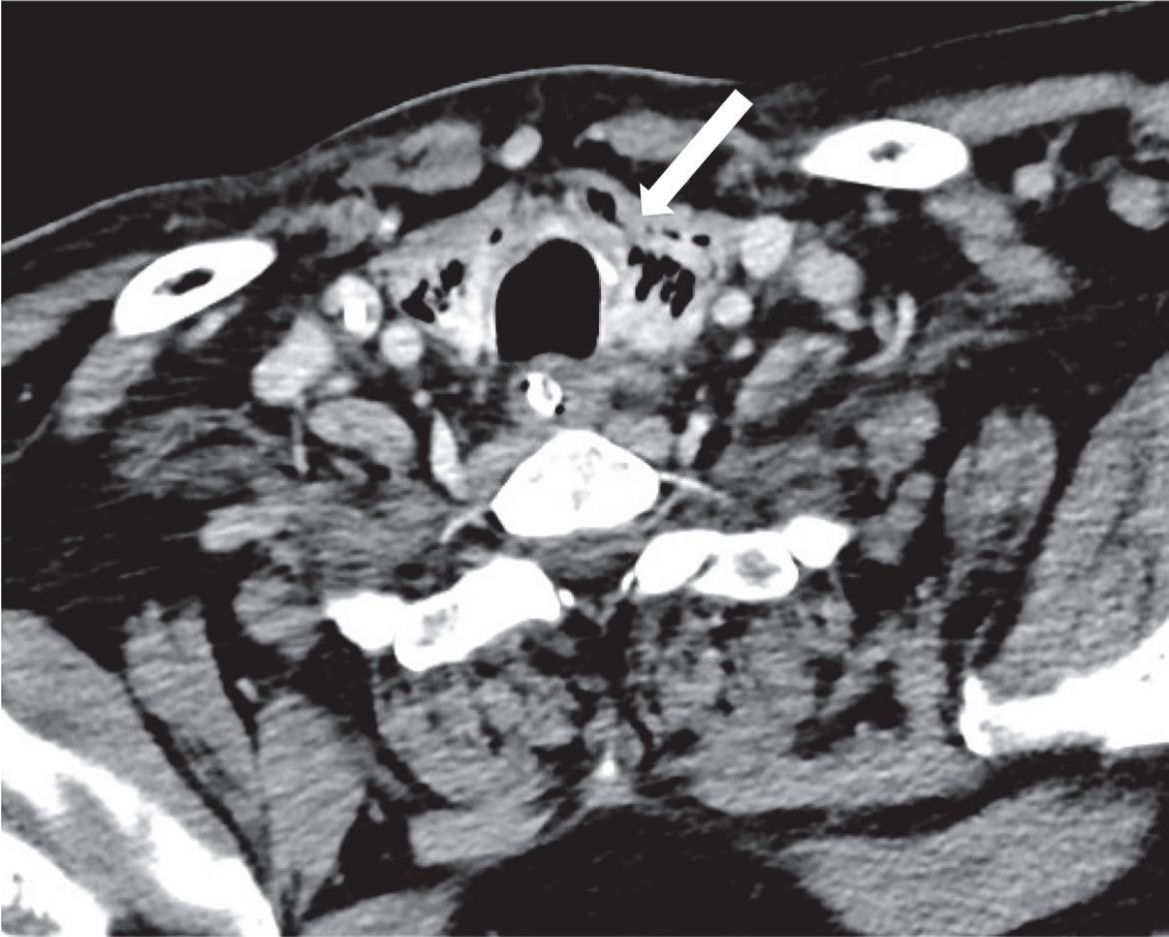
Axial image of IV contrast‐enhanced neck CT (1.50 mm slice thickness, contrast‐enhanced neck CT, venous phase, and 70 s), showing multiple gas bubbles within the thyroid parenchyma (arrow).
